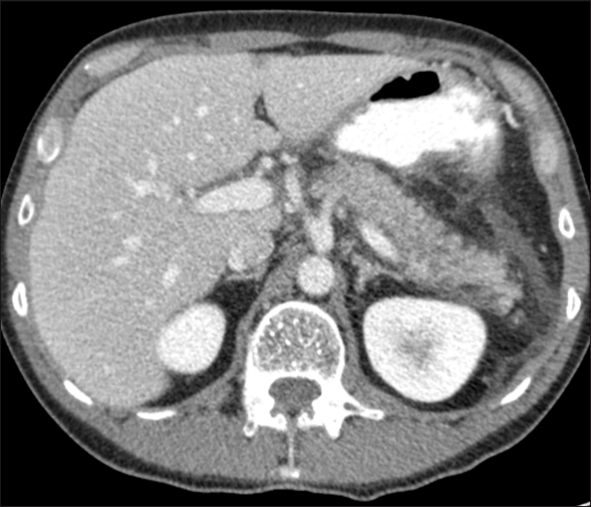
Contrast-enhanced axial CT scan (November 2006) showed edematous aspect of the pancreatic tail parenchyma with fluid in the anterior prerenal space, and in the anterior and posterior renal fascia.
